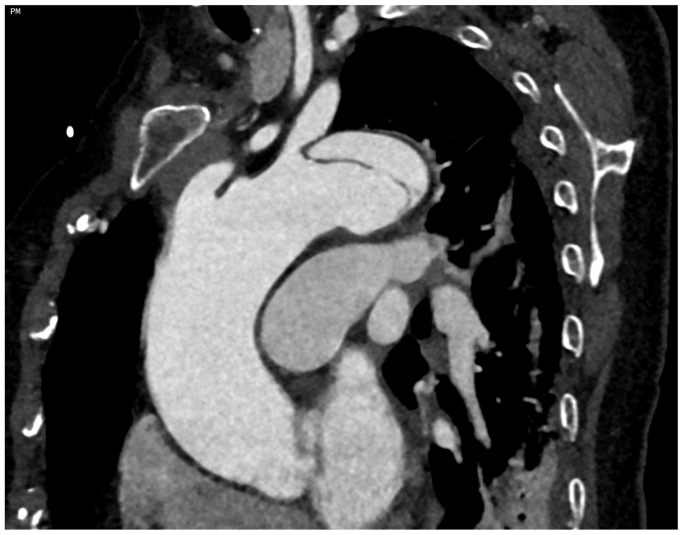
Computed tomography angiography (CTA) showing the type B dissection before endovascular treatment, with the location of the primary entry tear distal to the left subclavian artery (LSA).
